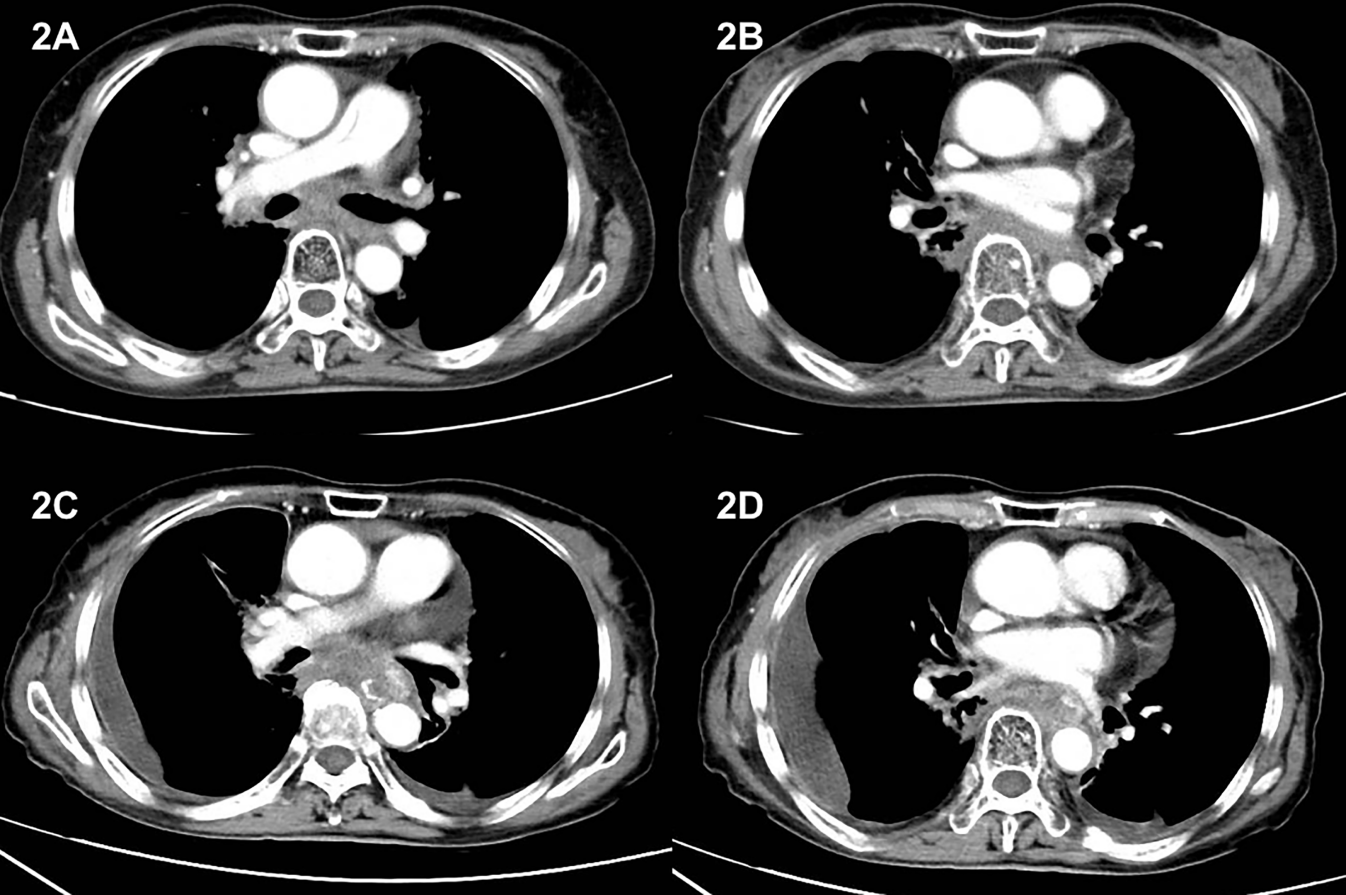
CT image during anlotinib treatment and after disease progression. (A, B) CT obtained on 3 March 2019, 4 months after initiating anlotinib treatment. Images showed soft tissue density mass in the posterior mediastinum and the range of the mass remained stable during anlotinib treatment. (C, D) CT image on 2 October 2020. Images showed enlarged posterior mediastinum mass, together with pleural effusion and pericardial effusion, indicating disease progression.
